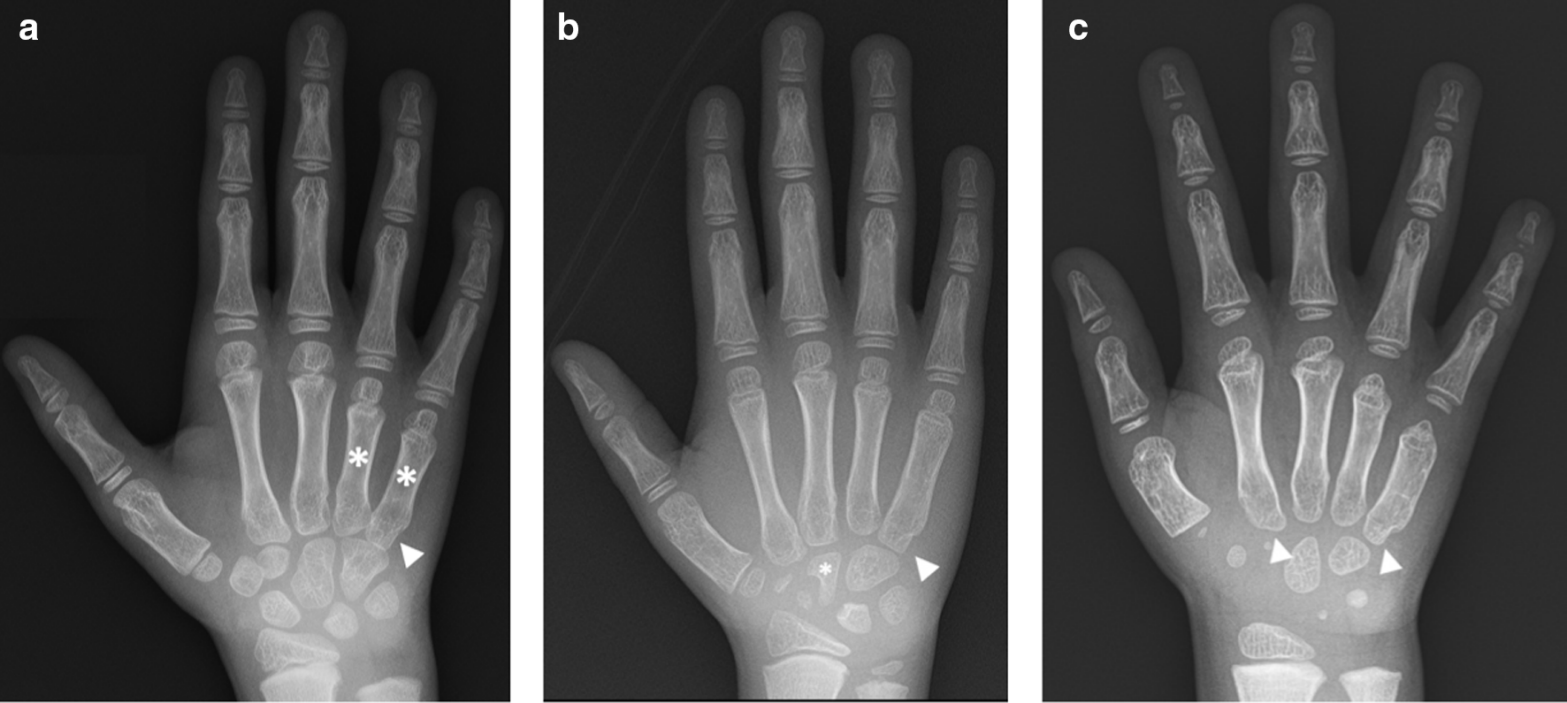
Radiographic features of the hand in the three siblings supportive of MPS IVA. a Patient 1 at 6 years of age showed prominent shortening of the fourth and fifth metacarpals (asterisks) with proximal pointing of the fifth metacarpal (arrowhead) and widening of the first metacarpal. b Patient 2 at 6 years of age showed proximal pointing of the fifth metacarpal (arrowhead) and irregular carpal bones (asterisk). Notably, this patient’s metacarpal abnormalities were much less conspicuous compared with those of his older brother (Patient 1) at the same age. c Patient 3 at 3 years of age showed proximally shortened, widened metacarpals with proximal pointing of the second and fifth metacarpals (arrowheads)
